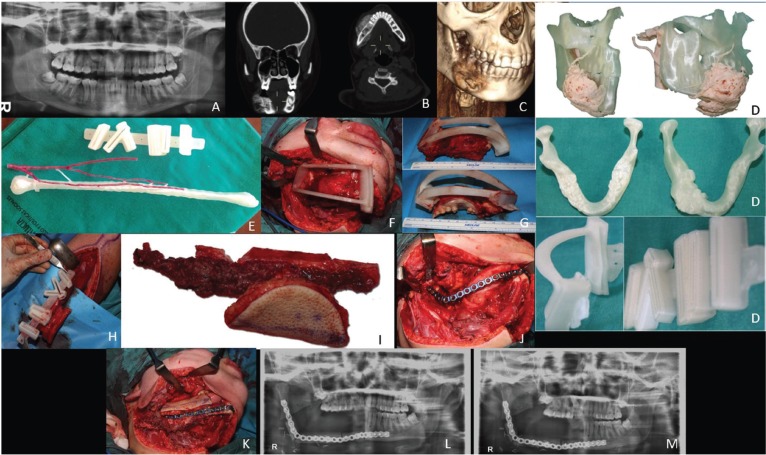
A - Preoperative orthopantomography. B - Coronal and transverse tomographic sections of the tumor lesion in the mandible. C - 3-D computed tomography of the tumor lesion. D - Manufactured surgical guides of the mandibular osteotomy and the fibula graft. E - A manufactured stereolithographic model of the fibula with its arterial vascularization and surgical guide for grafting. F - Positioning and intraoperative fixation of the surgical guide of the mandibular osteotomy. G - Surgical guide of the mandibular osteotomy after surgical resection of the tumoral lesion. H - Positioning and intraoperative fixation of the surgical fibula osteotomy guide. I - Multiple osteotomies of 2 to 3 cm in the osteomyocutaneous graft of the fibula. J - Positioning of the osteosynthesis plaque for the mandibular reconstruction. K - Mandibular reconstruction with free fibula graft segments. L - Postoperative orthopantomography two weeks after surgery. M - Postoperative orthopantomography six weeks after surgery.
